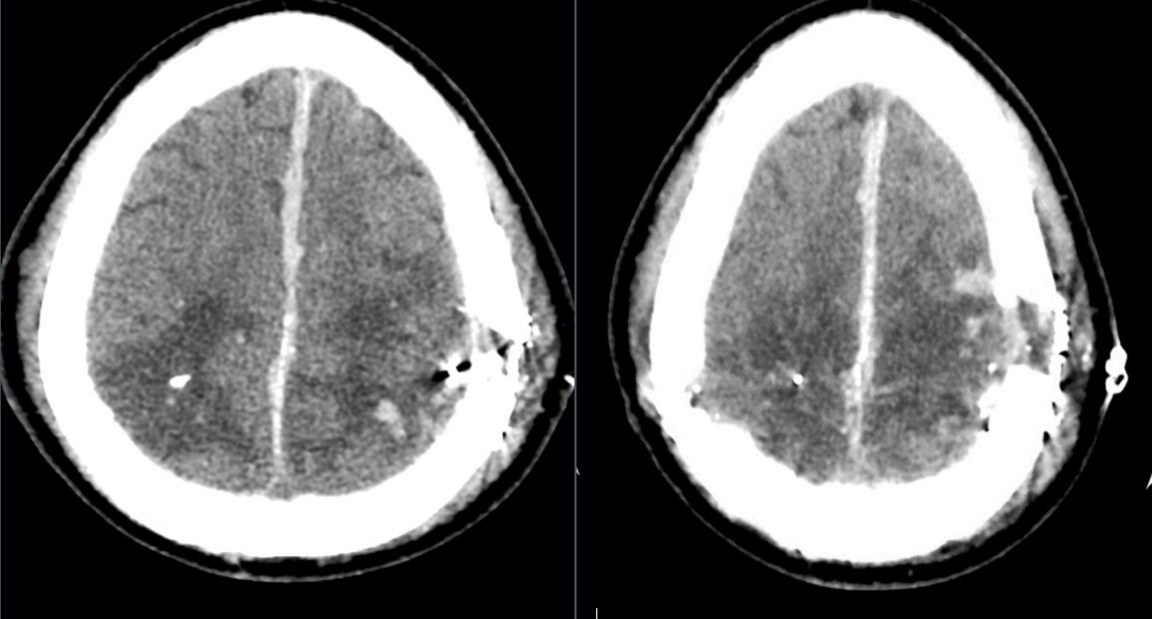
Post-operative computed tomography (CT) head revealed retained projectile and bone fragments along the trajectory. In addition, projectile fragments remained adjacent to the superior sagittal sinus which required anticoagulation due to risk of sinus thrombosis.
